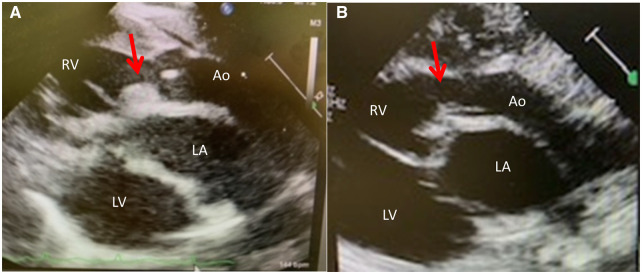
Echocardiography. (A) Preoperative: long-axis view showing right ventricular outflow tract stenosis. (B) Before definitive repair: long-axis view showing intact right ventricular outflow tract.
